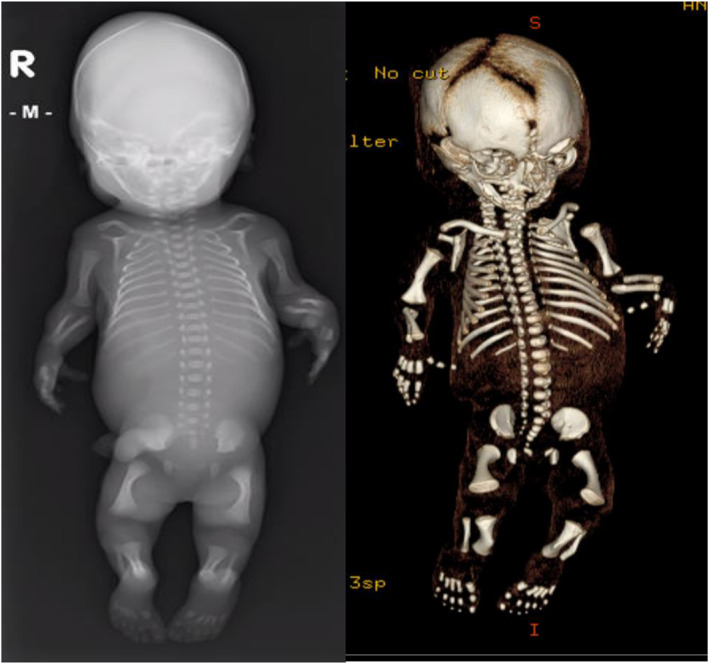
X‐ray and 3D‐CT of a terminated fetus (H20 + 6) with Diastrophic dysplasia. Long bones are short and wide and humeri and lower legs bowed. Wrists are flexed and ‘hitchhiker's thumbs' can be seen bilaterally.
